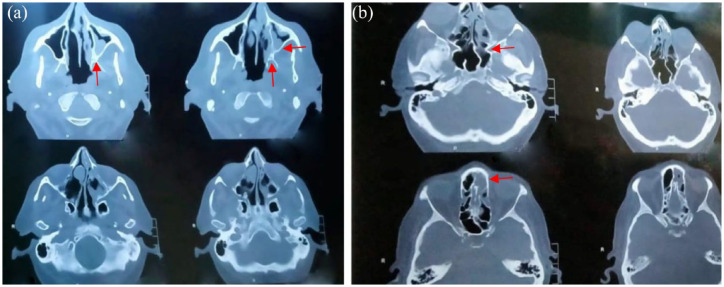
Contrast-enhanced computed tomography (CECT) images showing involvement of (a) maxillary and ethmoidal sinuses and (b) maxillary sinuses.
